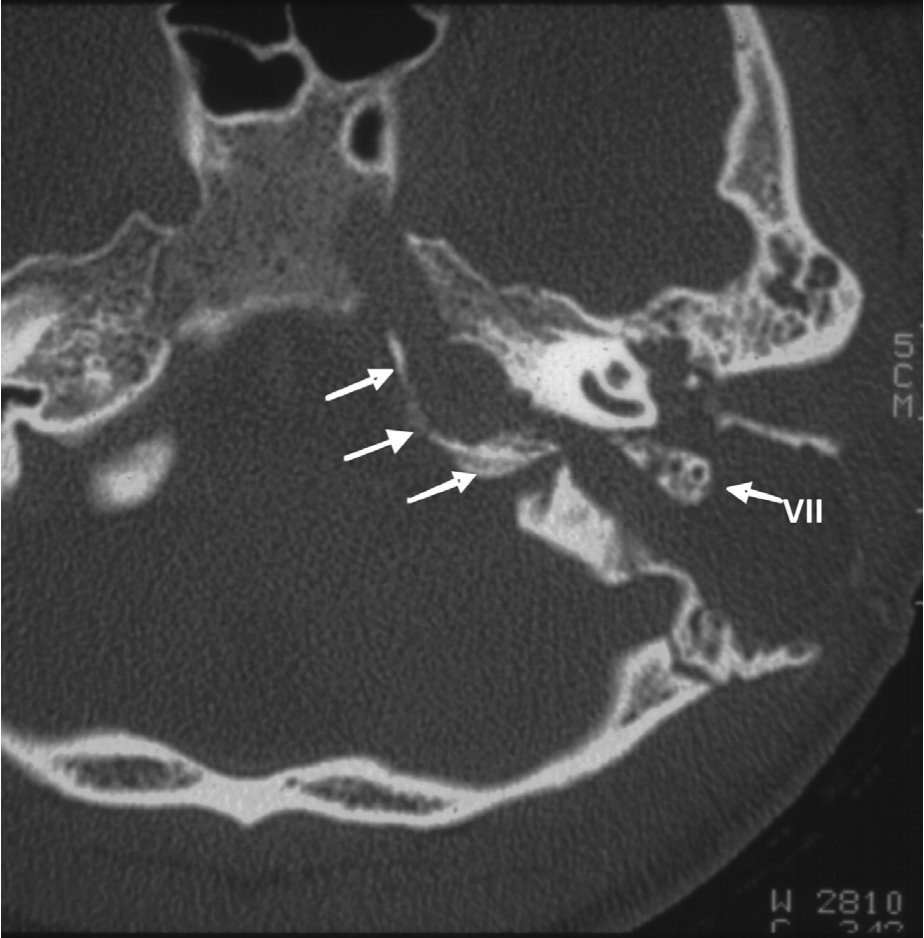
Postoperative computed tomography scan demonstrating the canal wall-up mastoidectomy and the path to the petrous apex (arrows). VII indicates the mastoid portion of the facial nerve.
